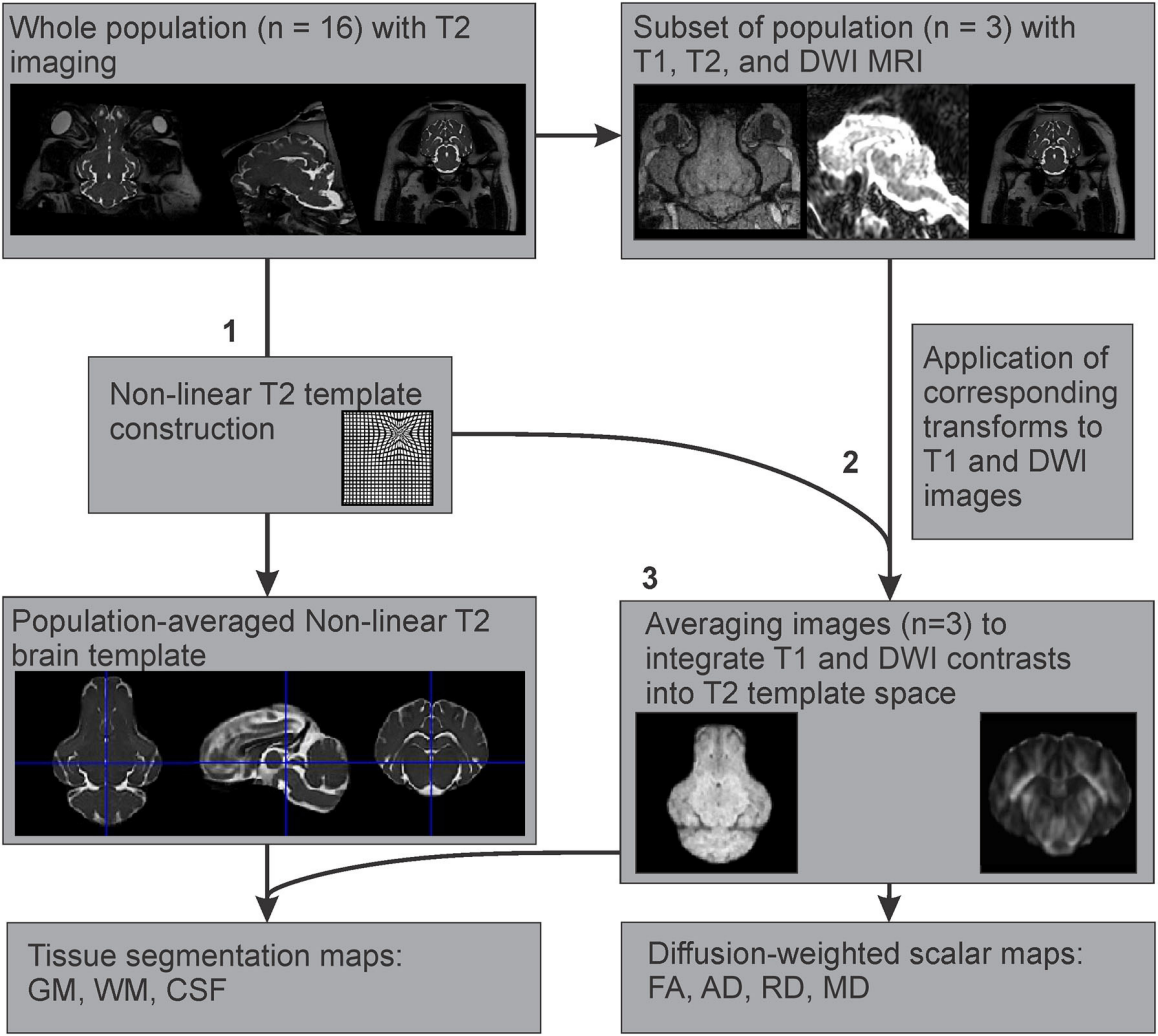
Multimodal registration of T1 and diffusion-weighted templates to the T2-weighted template space. After (1) creation of the population averaged T2 template, (2) the generated non-linear transformations were applied to the corresponding T1 and DWI images from a subset of the population where all three imaging modalities were acquired, to map their features onto the T2 template space. (3) These images (*n* = 3) were averaged to create T1 and DWI templates based on the T2 population template.
